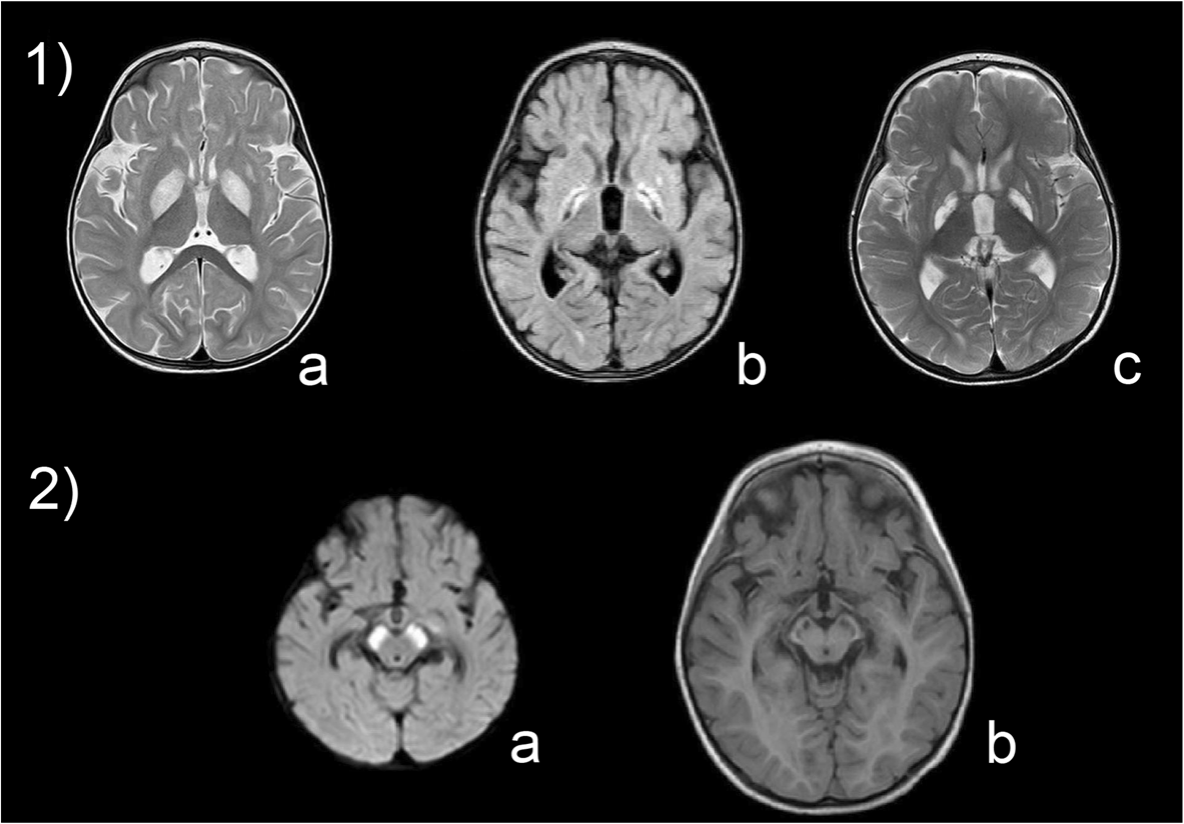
Brain MRI of patient 4. Brain MRI of patient 4 showing lesions in the basal ganglia (1) and mesencephalon (2). Bilateral hyperintensities are present in the globi pallidi and the left caudate at the age of 12 months (1a) and display progressive degenerative evolution to atrophy after 1 month (1b) and 6 months (1c) of the disease course. The substantia nigra shows acute lesions at 12 months of age (2a) and evolving into atrophy after 6 months (2b)
